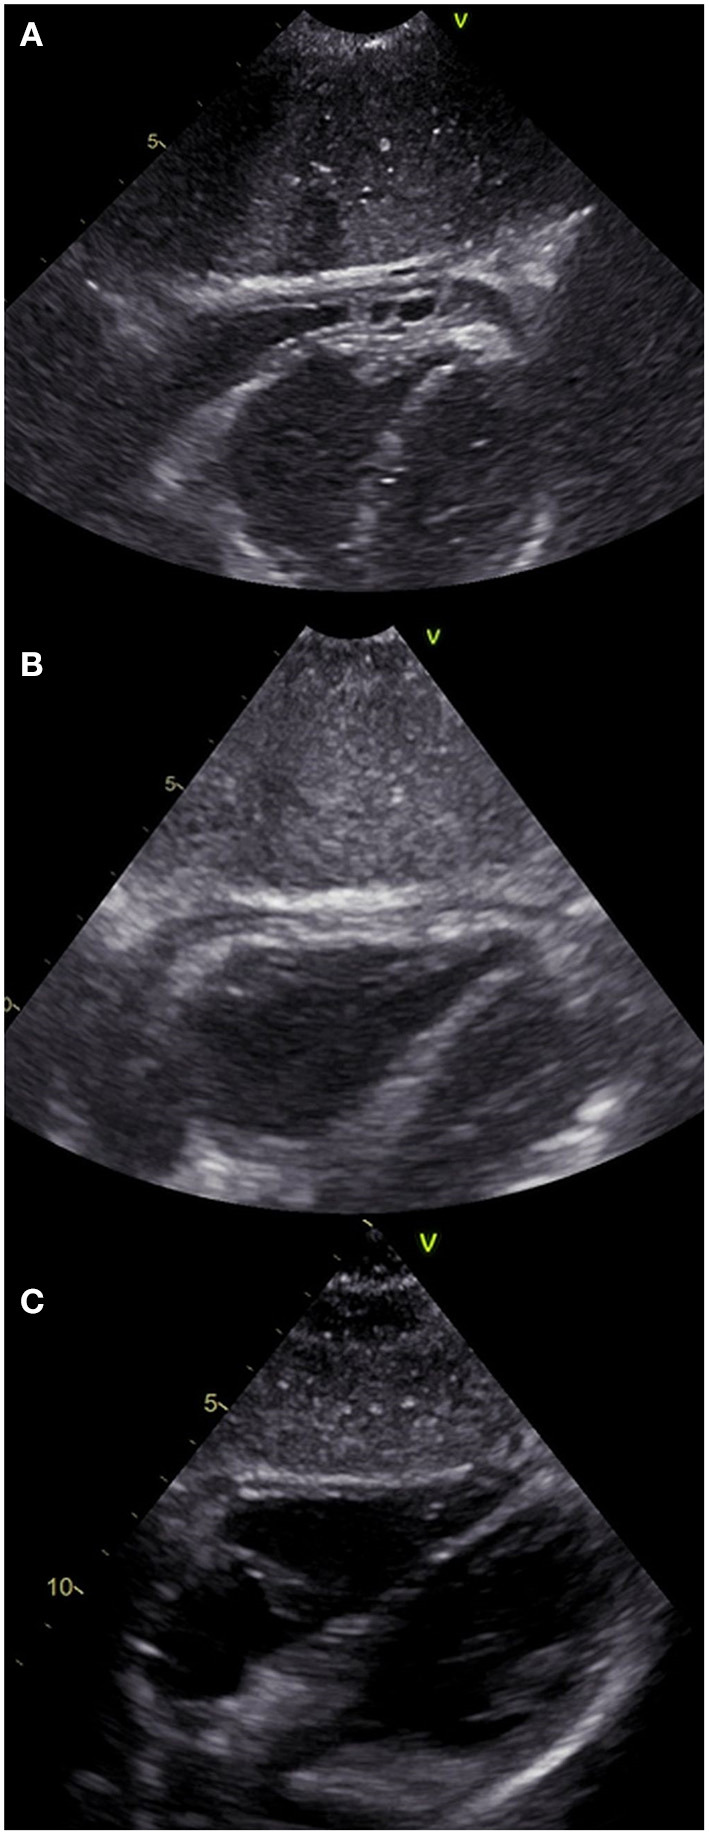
Echocardiographic subcostal view of the patient at different stages of the disease. (A) On admission to the Clinic of Pediatric Cardiology (2nd episode of fever). Both of the pericardial laminae are thickened with a pathological layer of fluid in between and fibrous adhesions in the apical region. (B) 7th day of hospitalization (4th day on prednisone). The laminae are still thickened, despite considerable reduction of the effusion. (C) 7 weeks after discharge showing normal physiological image.
